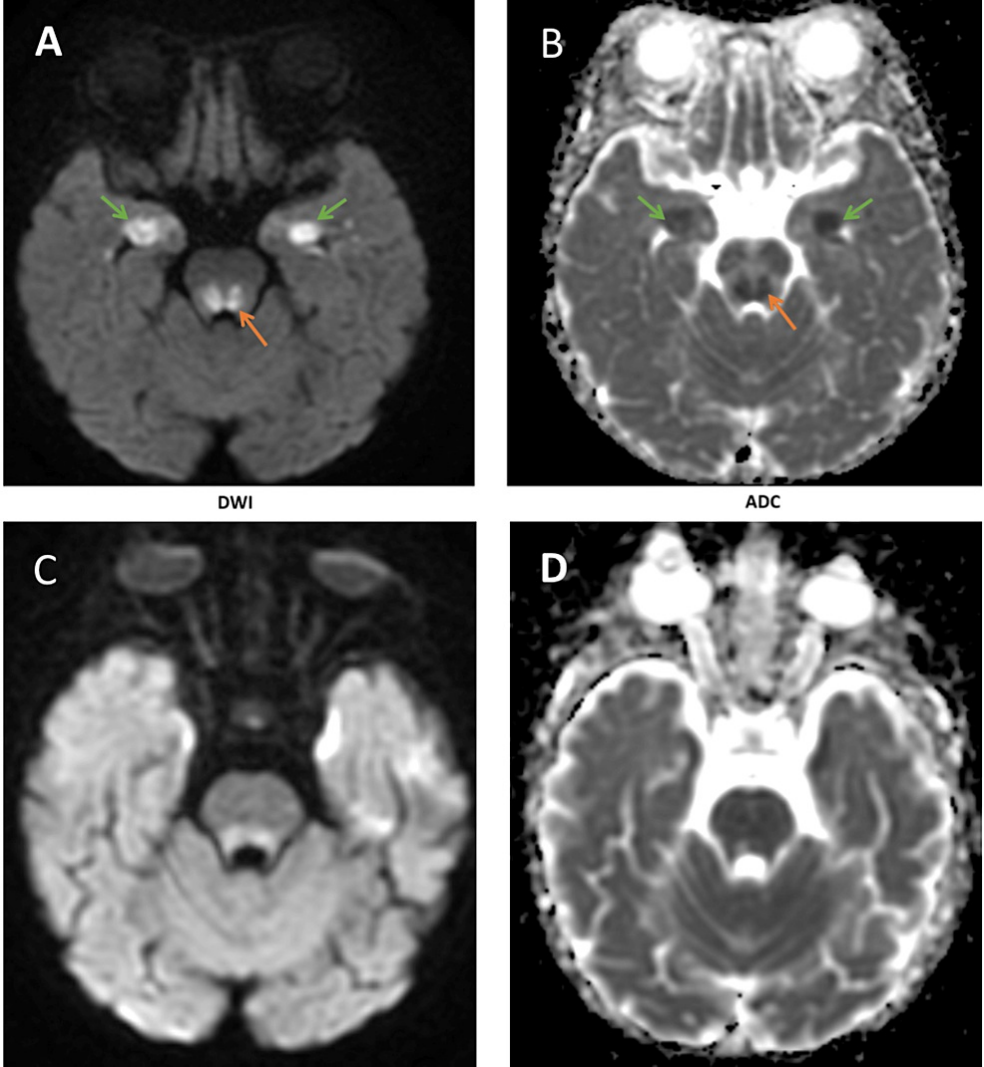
Restricted diffusion in the mesial temporal and dorsal pons Similar to the observation in Figure 3, there is restricted diffusion in the mesial temporal (green arrows) and dorsal pons (orange arrows). The bright spots on the axial DWI modality (A) image appeared dark in the ADC modality (B) image, which eventually resolved in both the DWI modality (C) and ADC modality (D) images. DWI: diffusion-weighted imaging, ADC: apparent diffusion coefficient.
